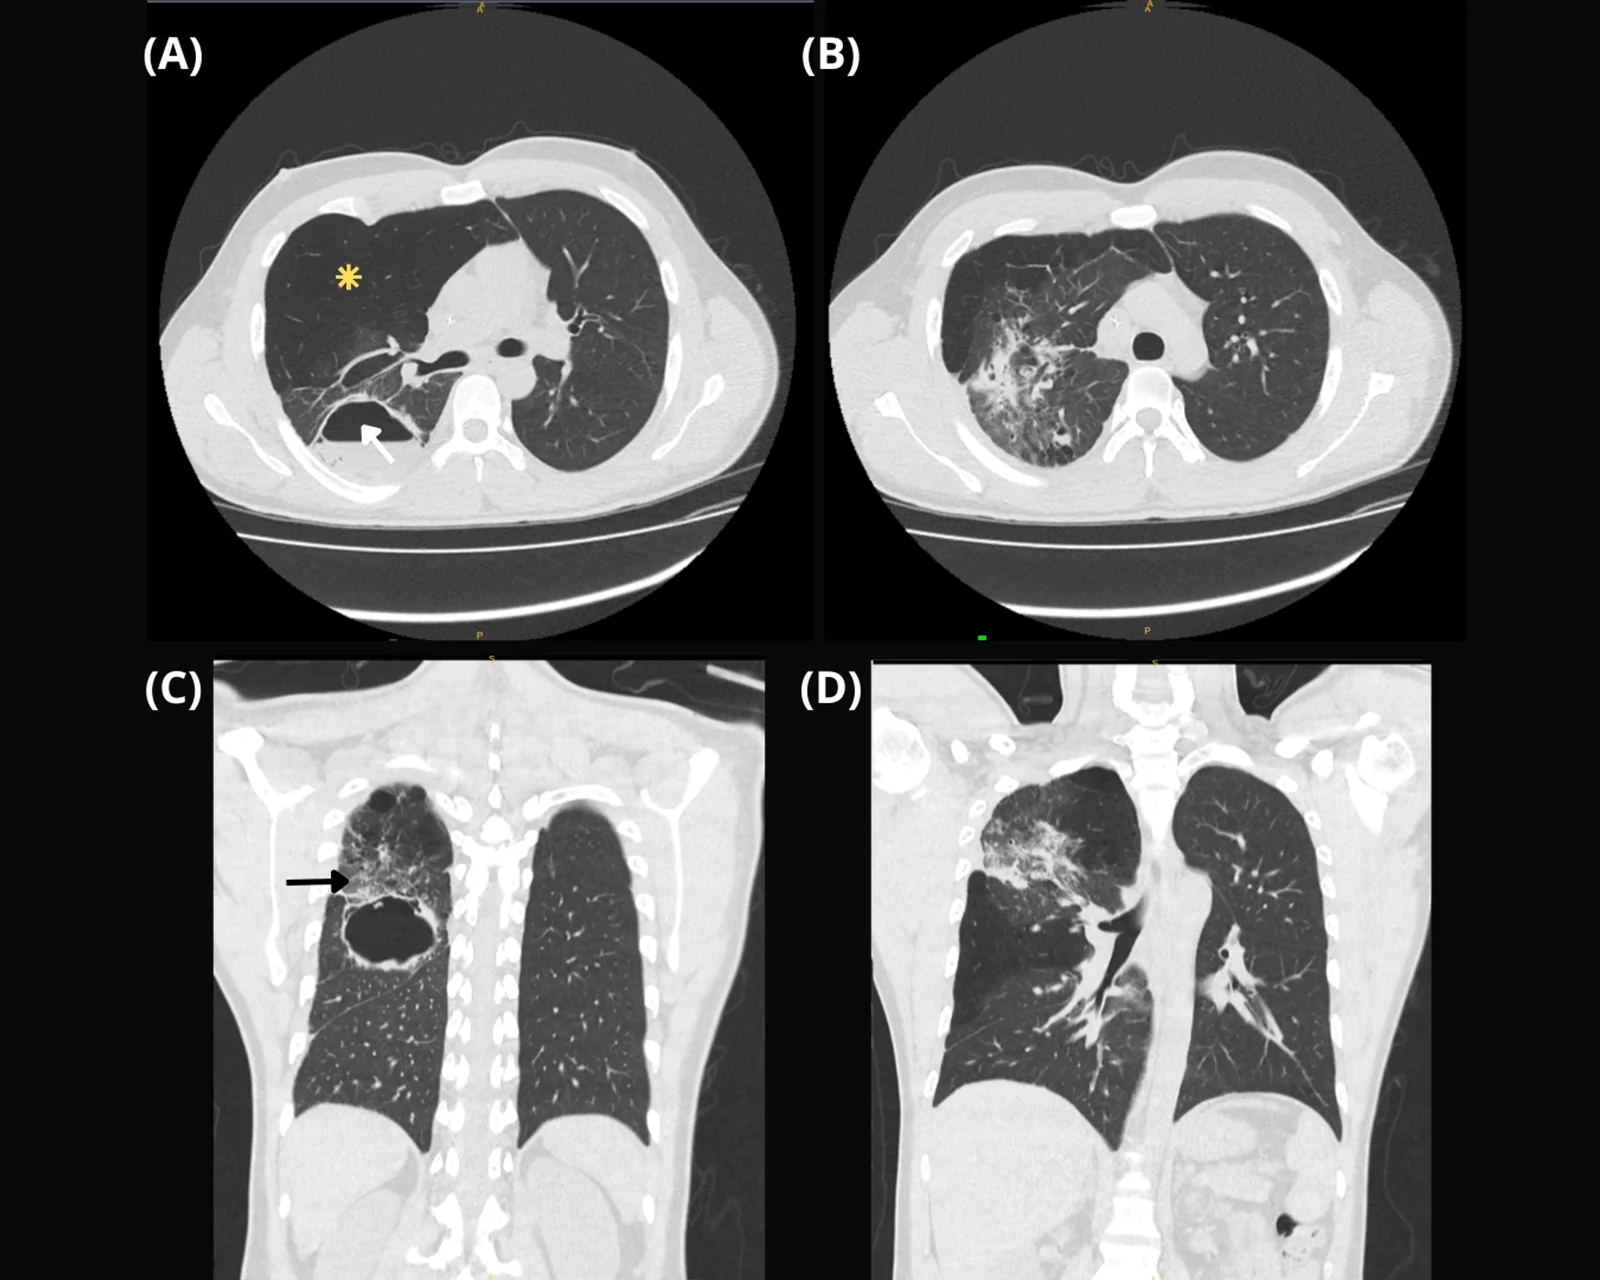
Follow-up contrast-enhanced chest computed tomography after completion of antibiotic therapy. Axial (A, B) and coronal (C, D) images demonstrate partial resolution of the right upper lobe consolidation (black arrow), persistence of a thin-walled cavitary lesion (white arrow), and persistent hyperinflation of the right middle lobe (*).
